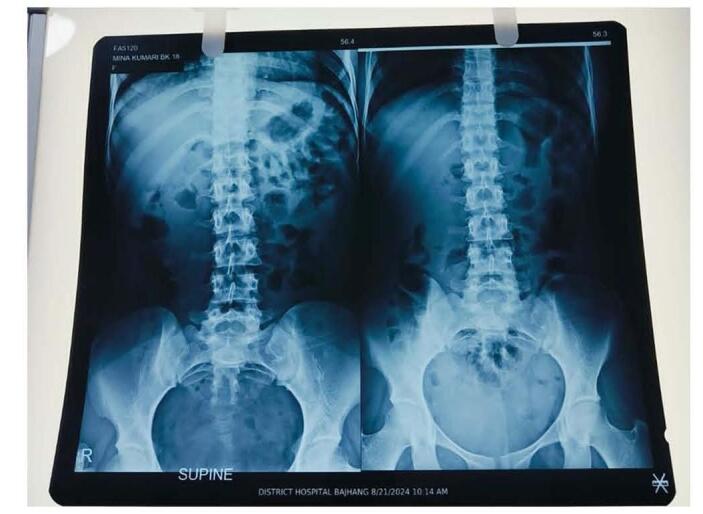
Post treatment X-ray abdomen erect and supine views showing no abnormality.
